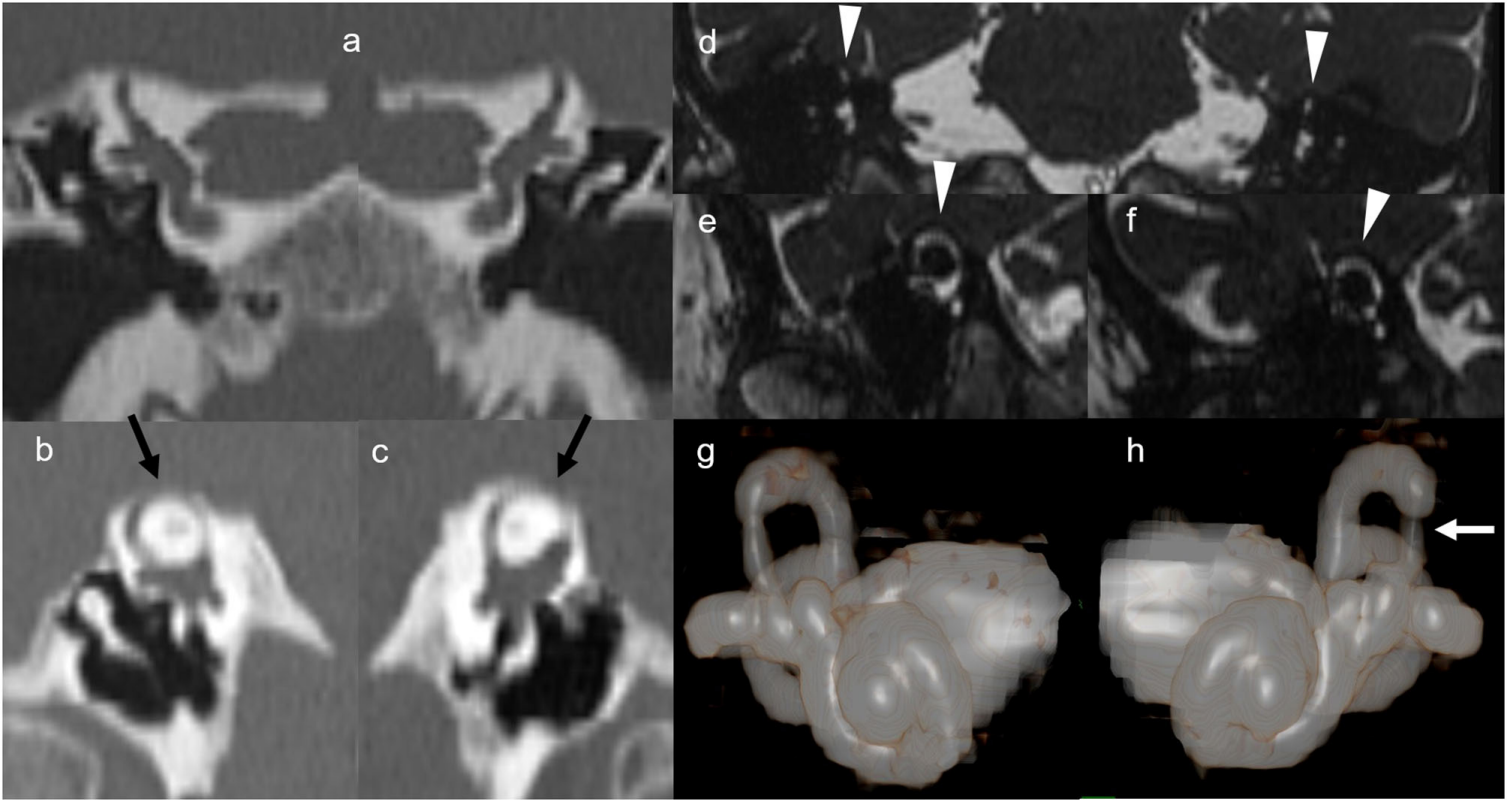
High-resolution computed tomography and magnetic resonance imaging. Multidetector computed tomography 1.0 mm-collimated coronal (a), and 0.5-mm collimated right (b) and left (c) sagittal oblique (so called Poschl plane) reformatted images obtained at admission in the emergency unit. Images according to the Poschl plane clearly show 5.5 and 3.5 mm-wide dehiscence of the bone overlying the right and the left superior semicircular canals, respectively (black arrows). These findings are consistent with clinical and audiometry findings. 1.5T magnetic resonance 3D-true fast imaging with steady-state free precession coronal (d), right (e), and left (f) sagittal oblique reformatted images obtained 2 months before as outpatient did not show the dehiscence (arrowheads). Note that right (g) and left (h) magnetic resonance 3D volume rendering anterior views shows thinning of both the lateral crus of both the superior semicircular canals, mainly in the left side (white arrow).
